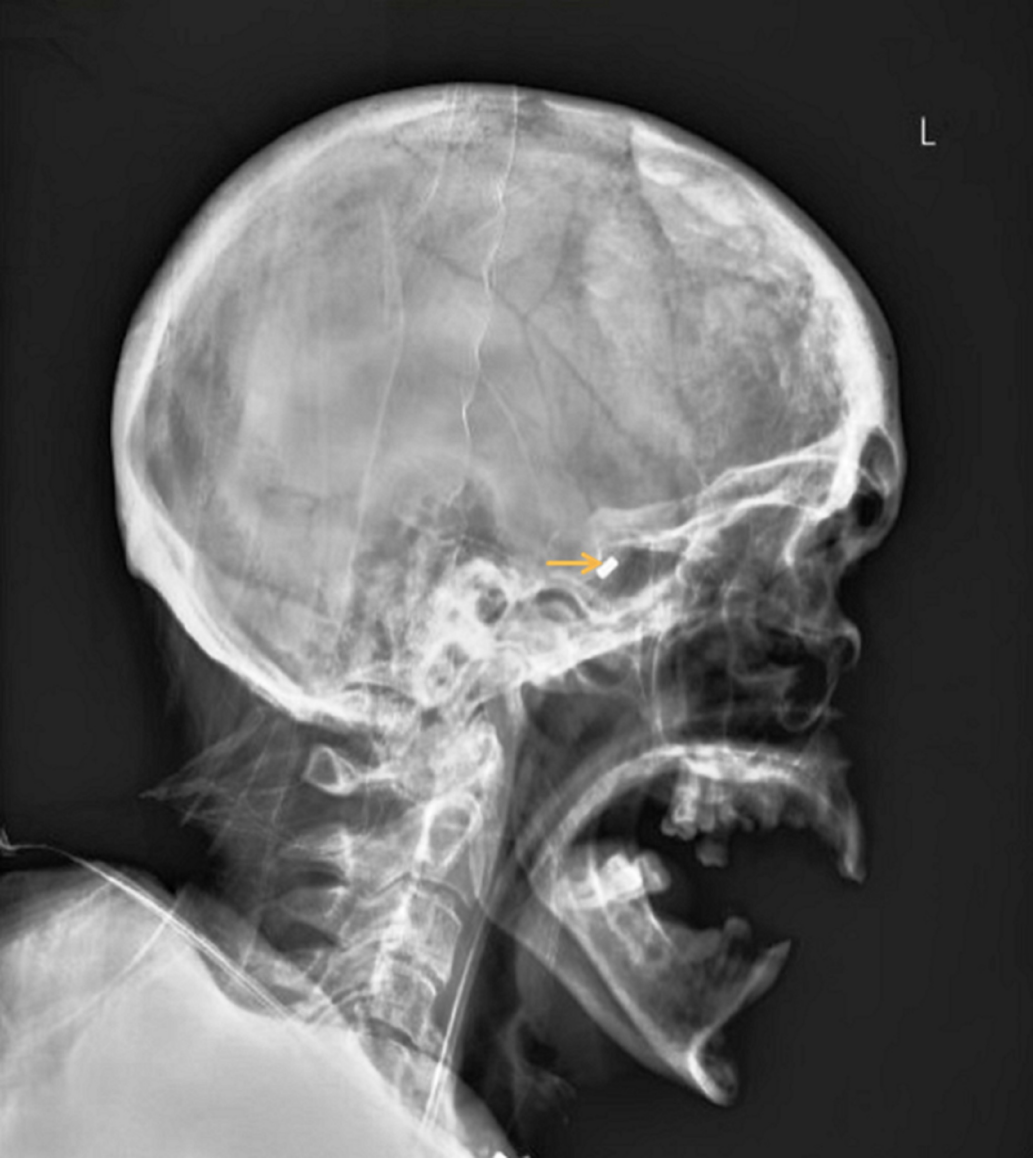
X-ray lateral view of paranasal sinuses showing a radiopaque object (arrow) lodged at the sphenoid os.
